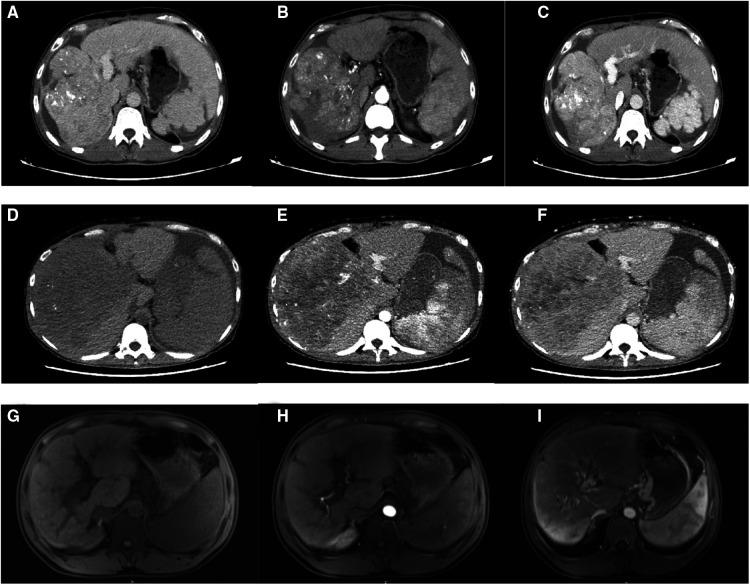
Postoperative CT images of case 1: unenhanced phase (A), arterial phase (B), and venous phase (C); postoperative CT images of case 2: unenhanced phase (D), arterial phase (E), and venous phase (F); and postoperative MRI images of case 3: unenhanced phase (G), arterial phase (H), and venous phase (I).
